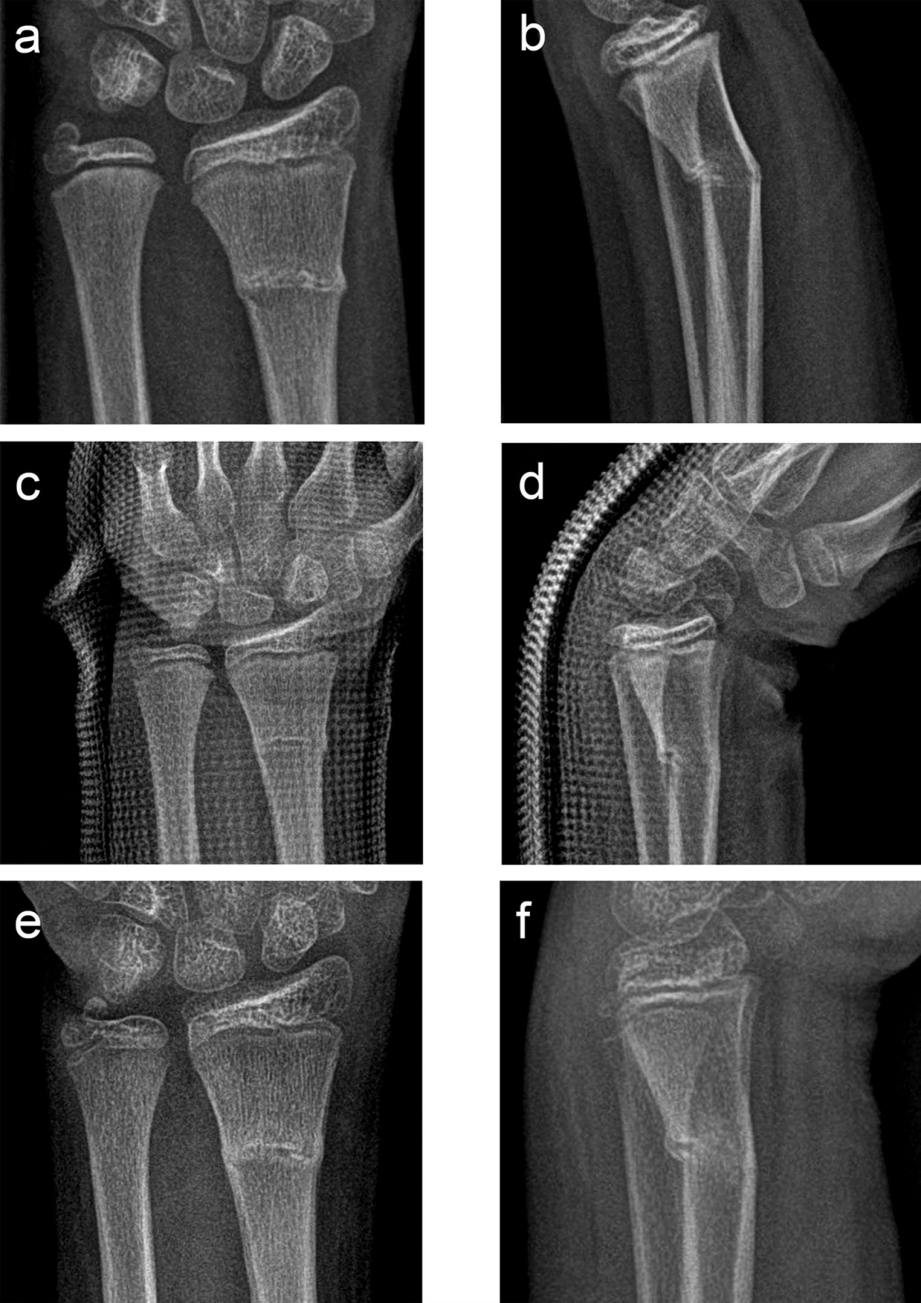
Ten-year-old female patient with a distal metaphyseal transverse fracture of the radius and an avulsion fracture of the ulnar styloid process on the left side following a fall from a swing (a, b). Radiographic alignment control on day 7 after reduction and cast application in a Schede cast under analgosedation (c, d). Radiographic consolidation control on day 27 followed by clearance for spontaneous mobilization (e, f). Clearance for sports activities was granted on day 50 after an unremarkable final clinical examination (no radiographic follow-up)
